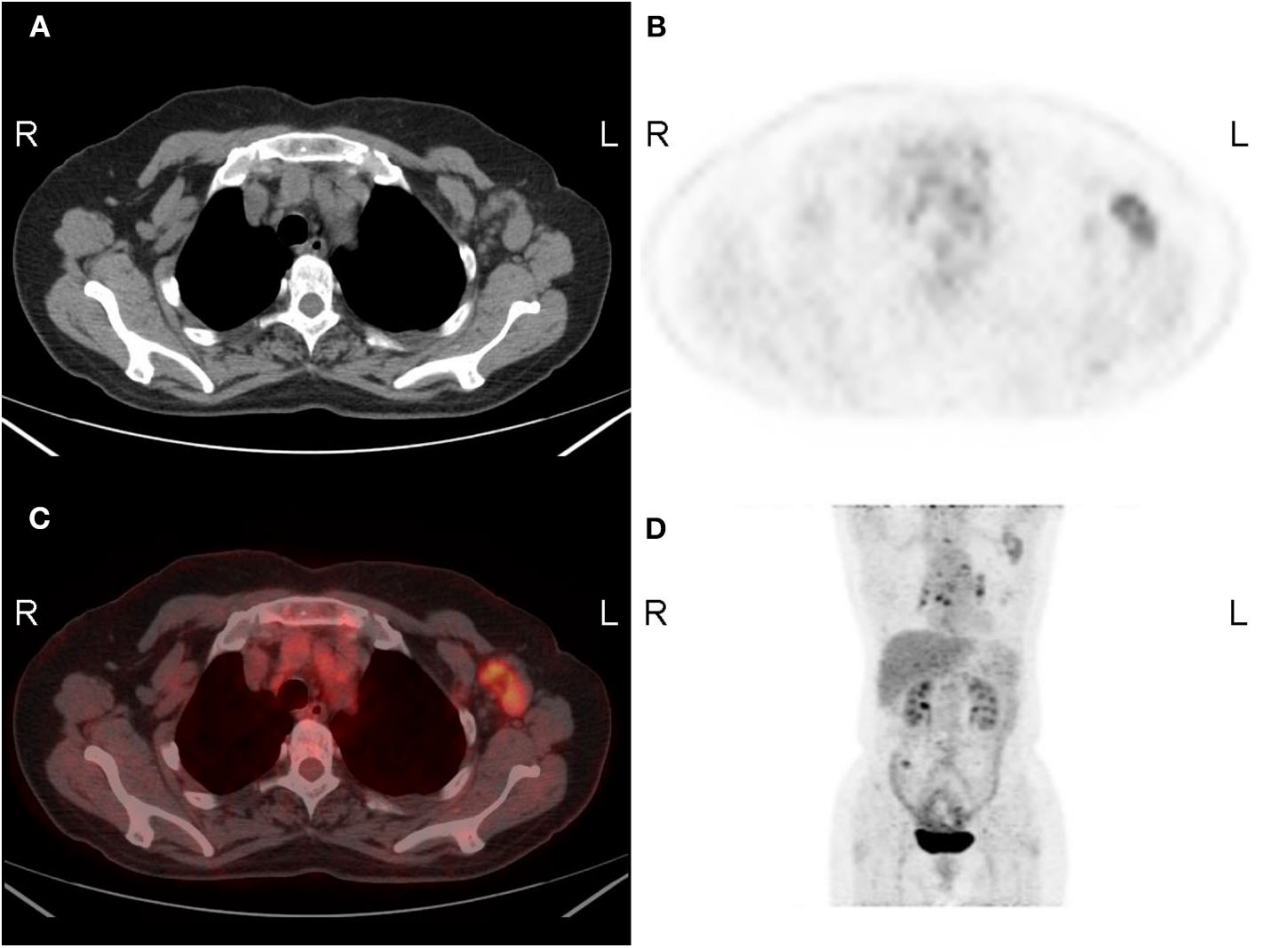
A 66-year-old woman with lymph-node metastasis of the left breast. Axial (A) CT, (B) FDG-PET, (C) fused image, and (D) MIP image show enlarged lymph nodes with intense uptake in the left axilla. The SUVmax was 3.4. R, right; L, left.
